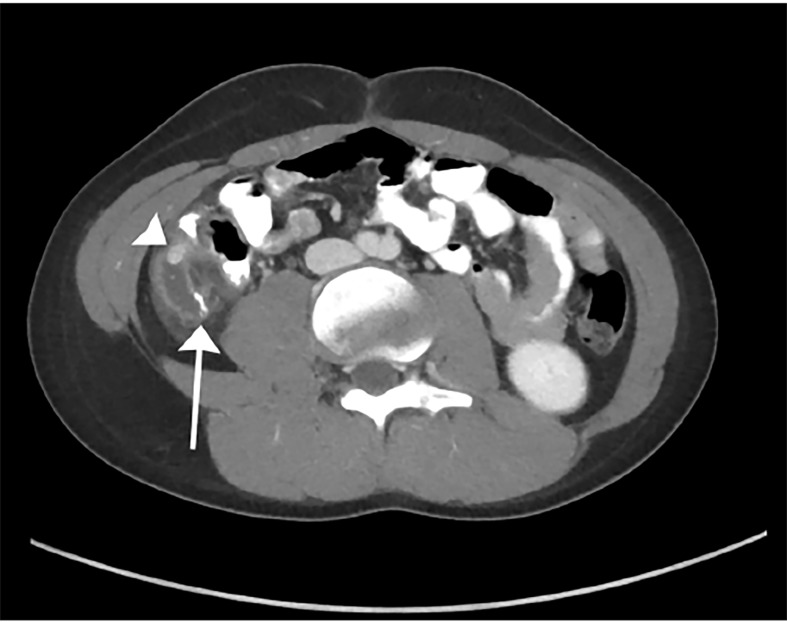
Contrast-enhanced computed tomography of the abdomen and pelvis, oblique axial plane, demonstrating surgical changes of appendectomy with staple lines at the blind end of the appendiceal stump (arrow). A high-density appendicolith (arrowhead) was obstructing the base of the appendiceal stump, which was surrounded by inflammatory changes.
